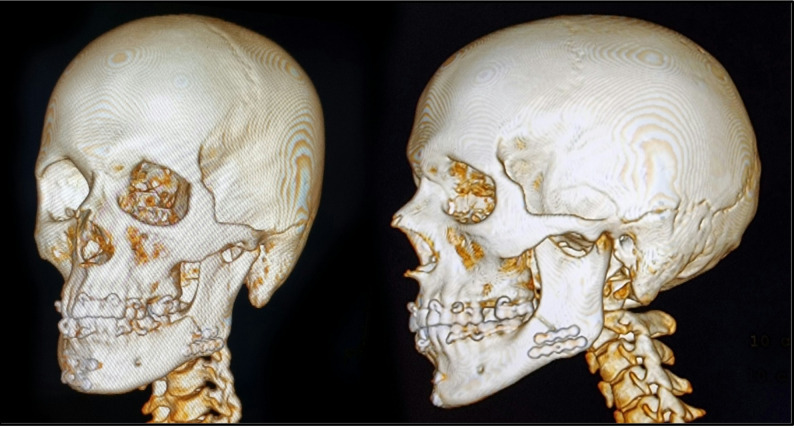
3D window of Postoperative CT view. CT images demonstrate adequate reduction and fixation of the fracture and precise anatomical alignment of the bone fragments with restoration of normal contour and continuity.
